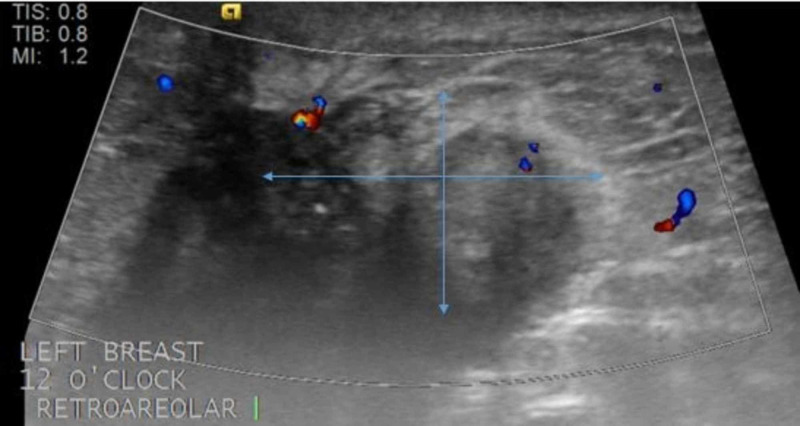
Ultrasound of the left breast in the transverse plane Targeted ultrasound demonstrates irregular mass with microlobulated margins measuring 27 x 17 x 21 mm at the site of the clinically palpable mass in the subareolar 12 o'clock region. Blue arrows outline the area of the mass
